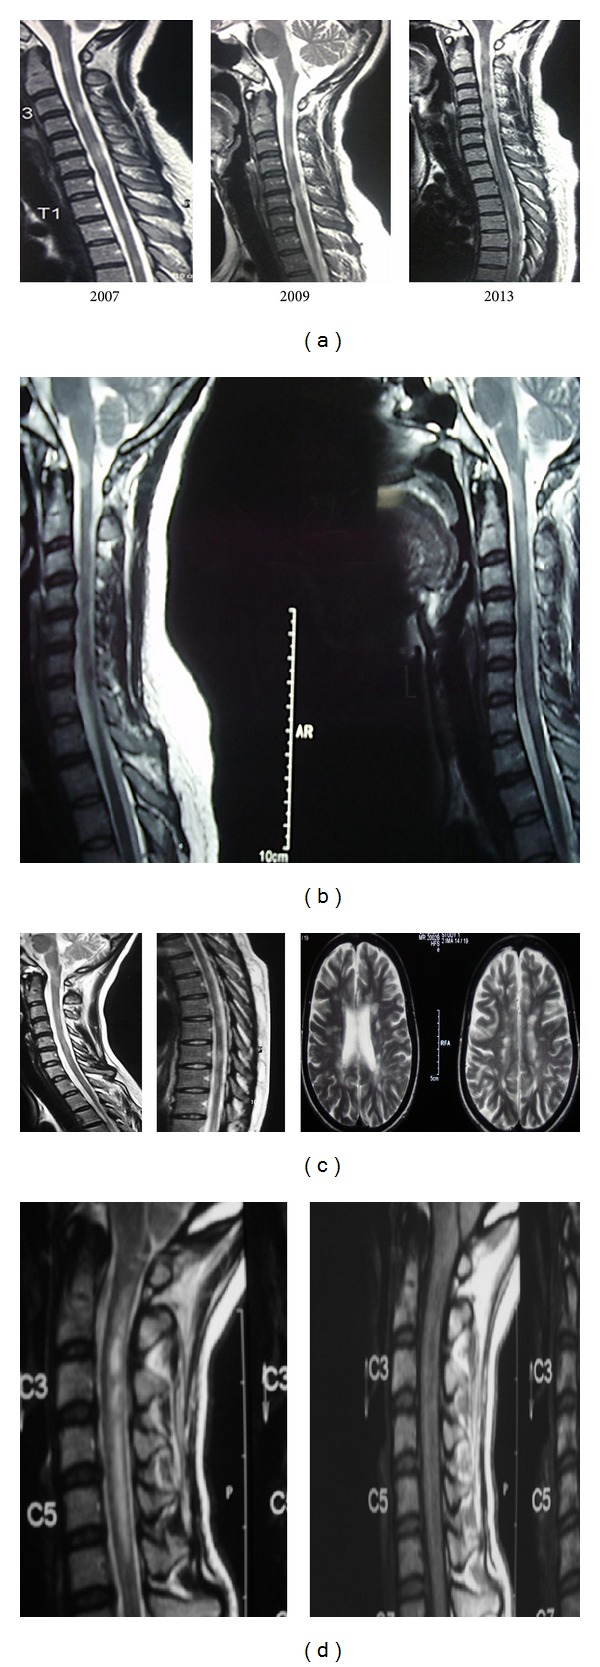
(a) Sagittal T2WI of the spine showing coalescing of multiple short segment lesions in a patient with relapsing-remitting multiple sclerosis at onset of disease and on follow-up once she entered the progressive phase of disease. (b) Sagittal T2WI of the spine showing a relapsing-remitting patient with multiple sclerosis with a short segment lesion superimposed on a longer patchy lesion at onset of disease. (c) Sagittal T2WI of the spine showing patchy ill-defined lesions more than 3 vertebral segments involving the entire cervicothoracic cord and axial T2WI of MRI brain with clinically definite multiple sclerosis in a patient with secondary progressive disease. (d) Sagittal T2WI (left) of the cervical spine showing a longitudinally extensive cord lesion with edema and corresponding T1WI (right) with T1 hypointensity within the cervical cord in a patient with neuromyelitis optica.
